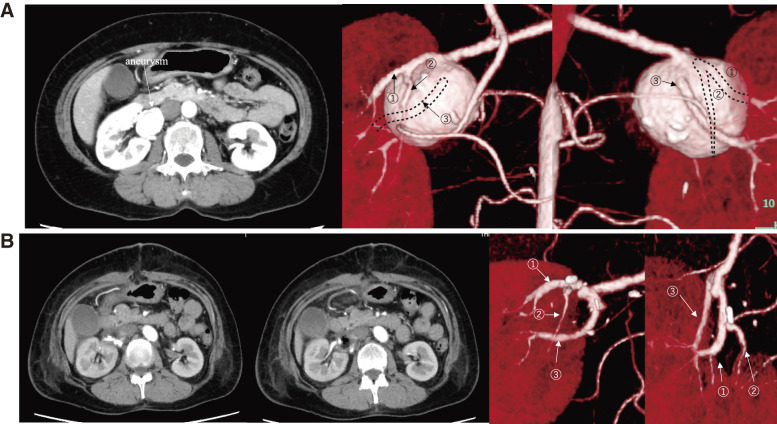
Fig. 1 Preoperative and postoperative CT. (A) The preoperative form of the renal aneurysm. The size of the renal aneurysm was about 32 × 35 mm (left picture). Middle and right 3D CT showed the following renal arterial flow: one inflow artery, two outflow arteries from the anterior to the aneurysm (numbers ①: superior segmental artery ②: anterior branch of the renal artery), and one outflow artery from the dorsal to the aneurysm (number ③: posterior branch of the renal artery). (B) postoperative CT after renal reconstruction. The axial view showed blood flow in the reconstructed artery could be confirmed. 3D CT confirmed that the outflow artery has been preserved. CT: computed tomography
